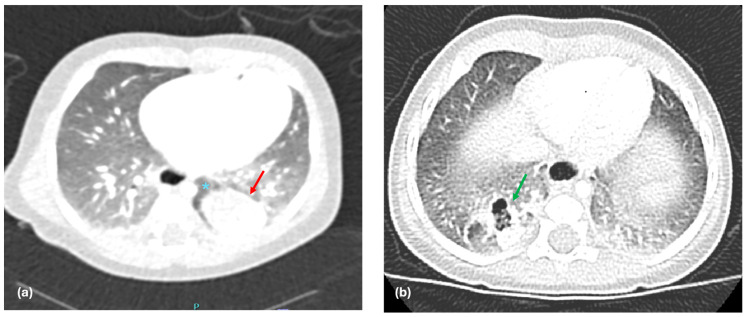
(a) CT scan showing a left lower ELS with sharp margins (red arrow: sharp margins of the lesion; blue asterisk: area of lung hyperinflation). (b) CT scan showing a right lower ILS with undefined margins (green arrow: undefined margins of the lesion).
